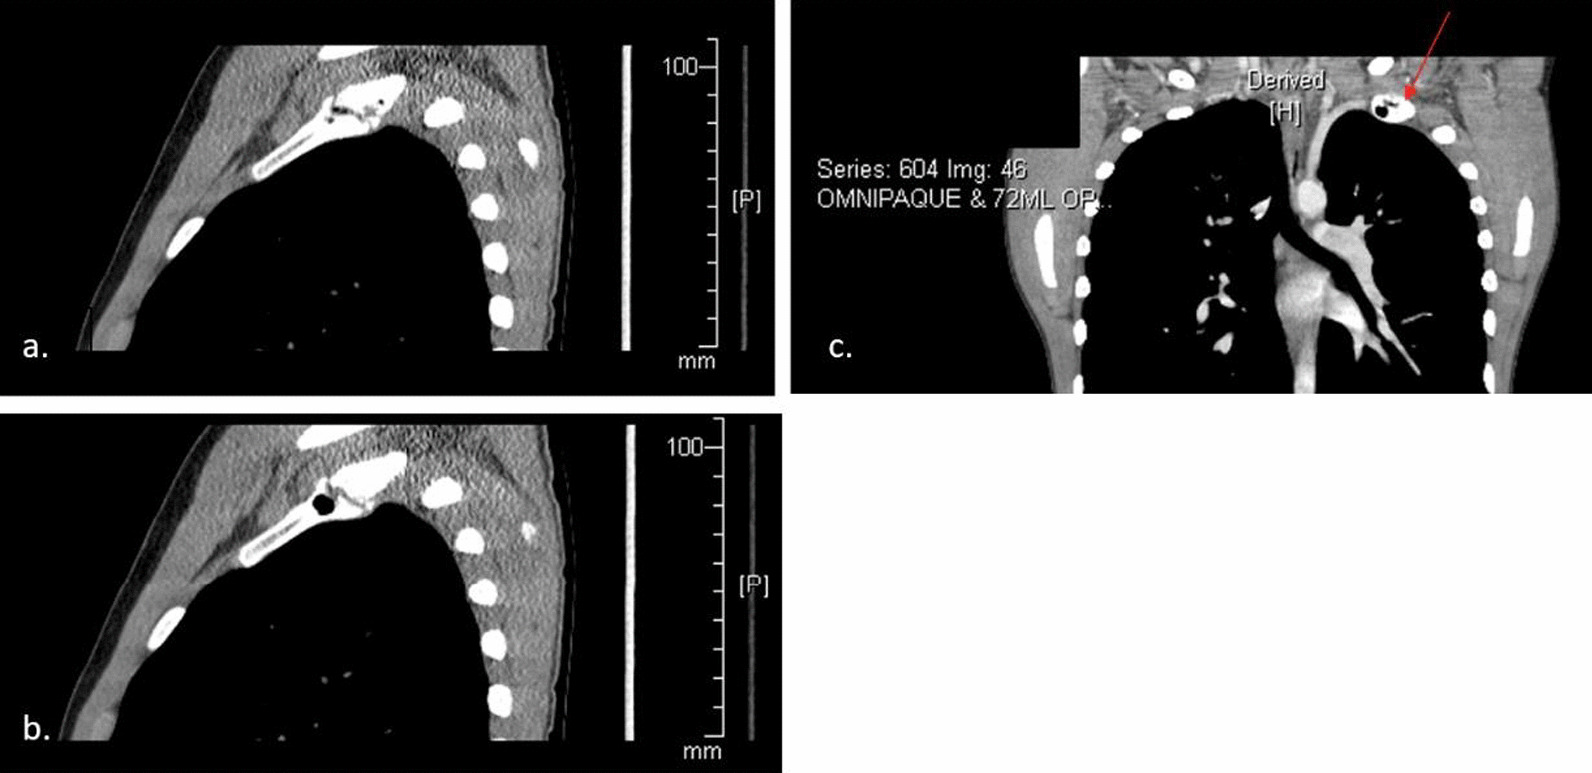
a, b sagittal computed tomography (CT) showing non union of first rib. c coronal CT exhibiting pseudoarthrosis of left first rib with vacuum phenomenon. The red arrow shows the accumulation of gas noted in the left first rib. This is demonstrating the pseudoarthrosis and vacuum phenomenon from the unhealed fracture
